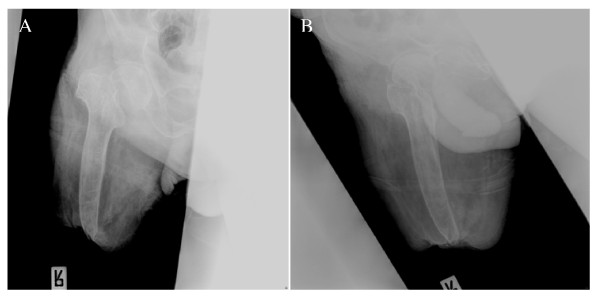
An anteroposterior (a) and lateral (b) xrays of the fracture.
